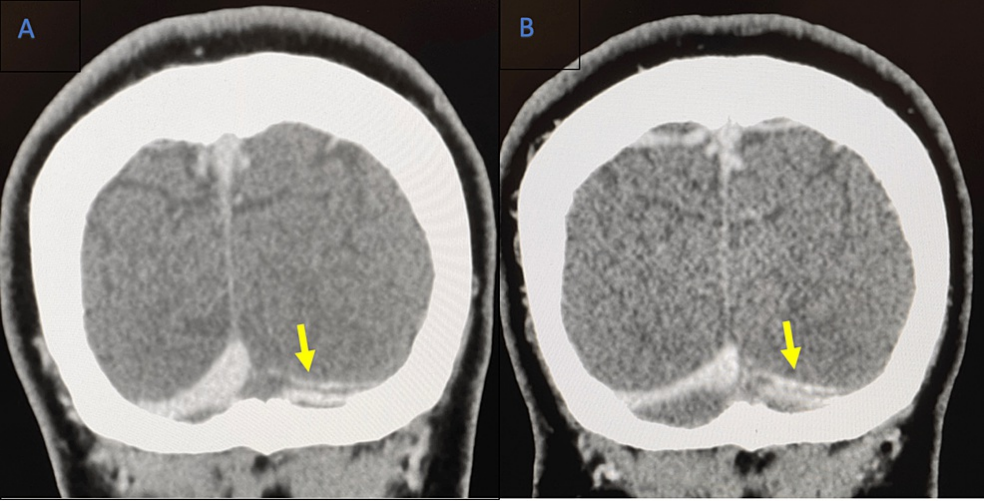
CT venogram demonstrating CVST in the left transverse sinus A: CT venogram at diagnosis, B: CT venogram six months after therapy.
